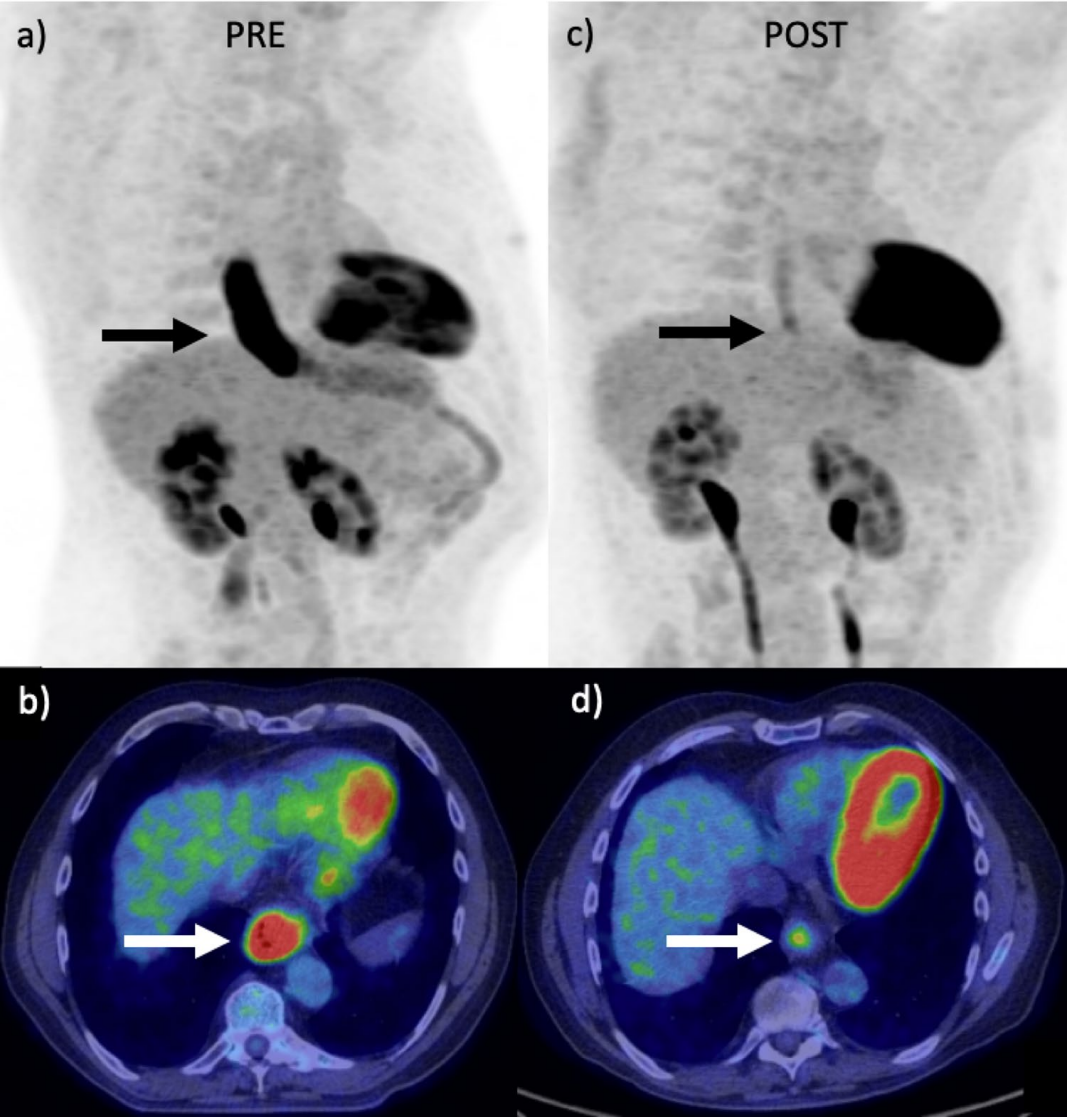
Selected images from a patient with a distal oesophageal adenocarcinoma. Maximum intensity projection (MIP) (a) and fused axial PET-CT images (b) demonstrated a large FDG-avid tumour. After neo-adjuvant chemotherapy was completed, a repeat PET-CT (c, d) showed there had been an excellent metabolic response. This patient had a positive DDIR assay and final pathological examination indicated a good response (tumour regression grade 2).
